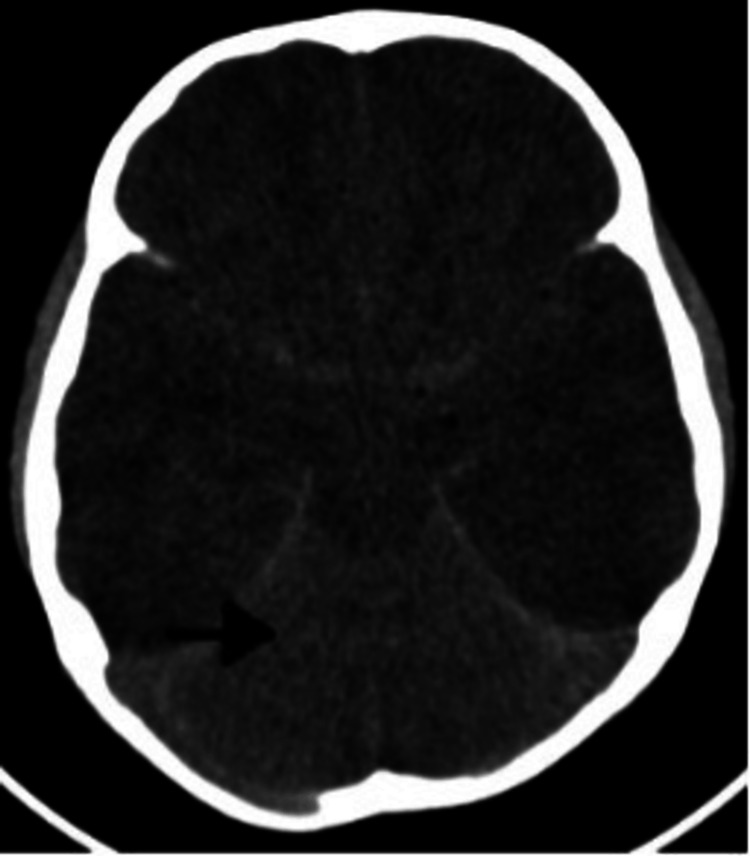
CT image of an H1N1 encephalitis patient in this study showing "white cerebellum sign," which is a poor prognostic indicator CT: computed tomography
